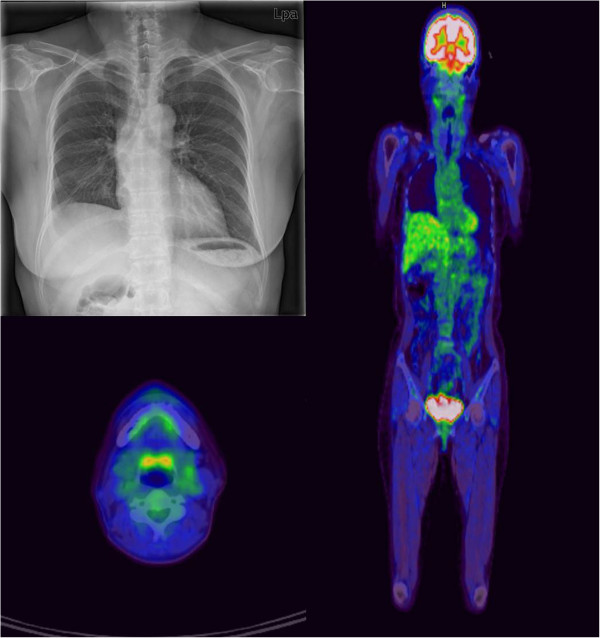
Chest X-ray and PET-CT after lobectomy show that the tumor from the salivary glands could not be detected clinically. The diagnosis made at that time was thus primary peripheral pulmonary ACC. ACC, adenoid cystic carcinoma; CT, computed tomography; PET, positron emission tomography.
